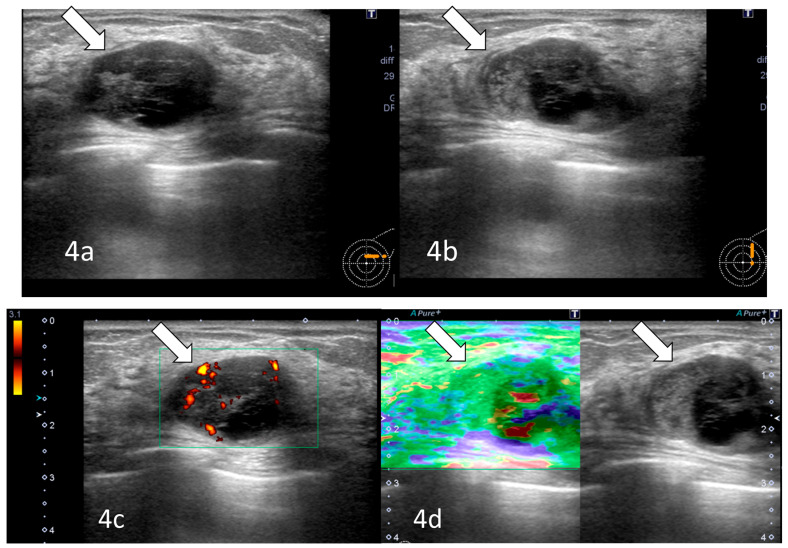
Ultrasonography (after 11 years): A 27 mm oval hypoechoic mass with central low and peripheral iso- to hyperechoic regions and posterior enhancement (arrows) was observed (a,b). Color Doppler imaging showed abundant peripheral flow (arrow) (c), and strain elastography showed mildly reduced strain (arrow) (d).
